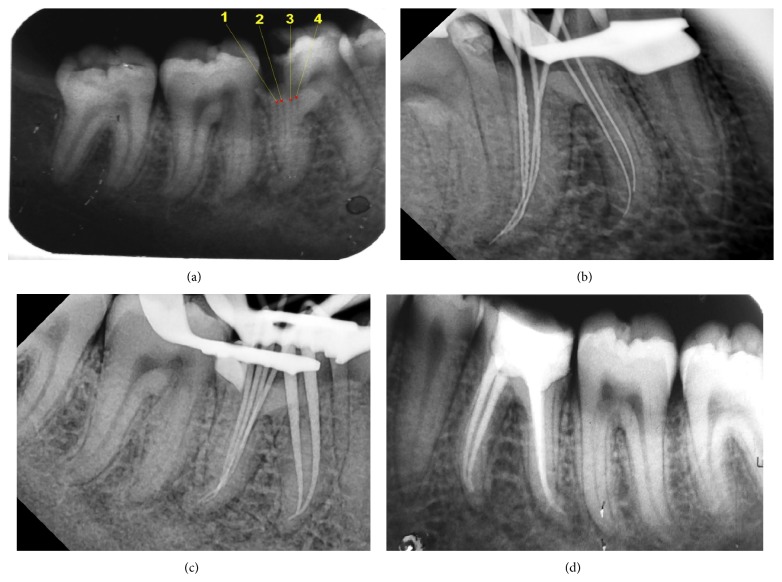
(a) Preoperative intraoral periapical radiograph 1-distobuccal I, 2-distobuccal II, 3-middistal, and 4-distolingual. (b) Radiograph showing working length determination of six root canals. (c) Master cone radiograph. (d) Postobturation radiograph.
